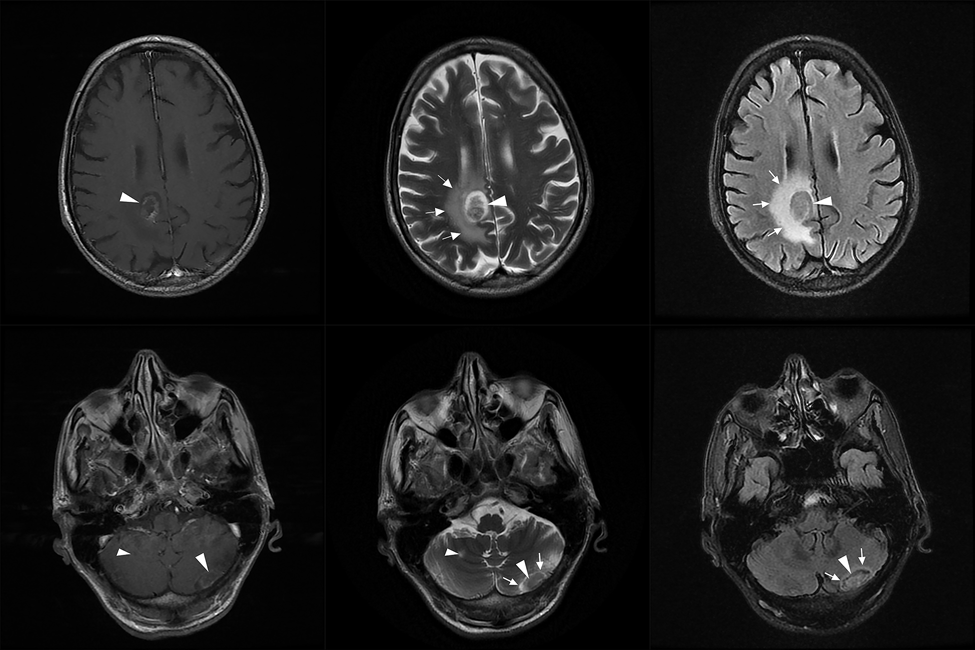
Brain MRI reveals a right sided mass (white arrowheads) in the corpus callosum with perilesional edema (white arrows) and mass effects on the ventricle, and two lesions (white arrowheads) in the hemispheres of cerebellum accompanied by edema (white arrows). [Left to right: T1 with gadolinium contrast, T2, and FLAIR views]
